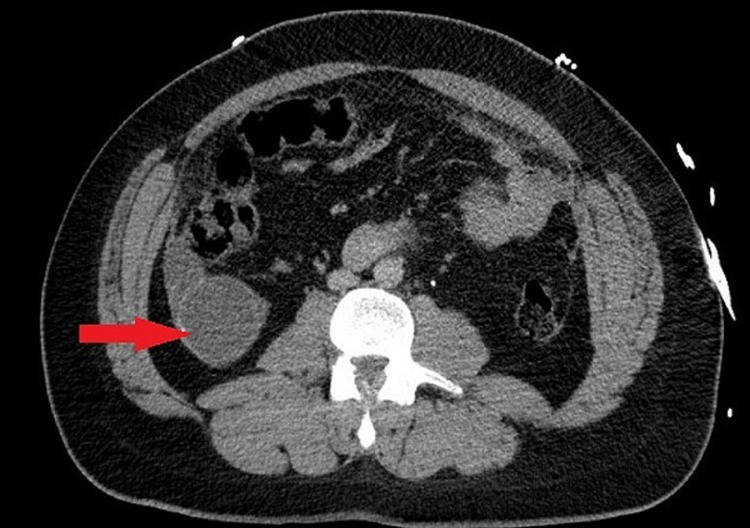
CT demonstrating 5.6 x 4.9 centimeter mass at the tip of the appendix.
